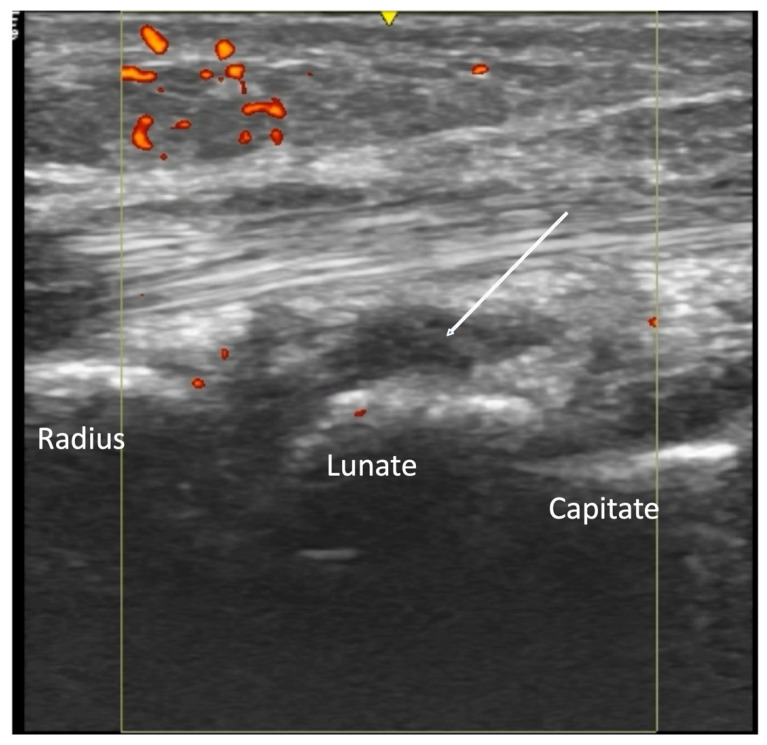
Noncompressible hypoechoic material (indicated by arrow) with no Doppler signal seen within the radiocarpal and intercarpal joint of the wrist.
